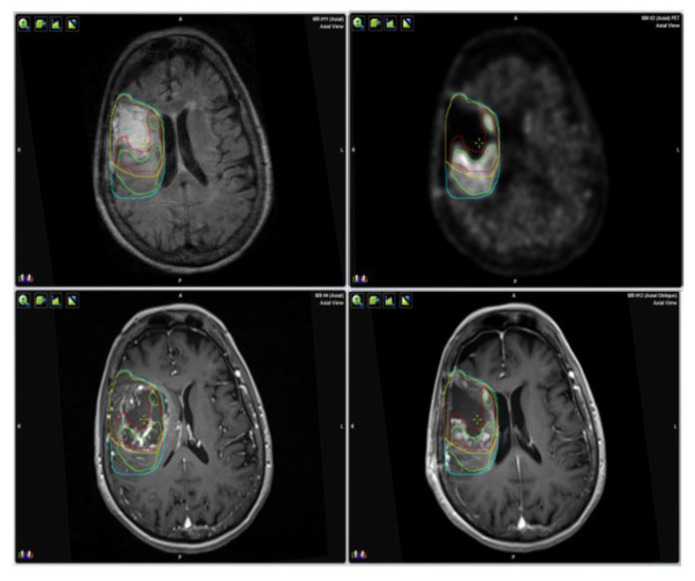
Volumes definition on post-contrast MRI, FLAIR, MRI and 11CMETPET. Clinical target volume 1 (CTV1)—yellow line; clinical target volume 2 (CTV2)—light blue; contrast-enhanced residual tumor volume (CERTV)—red; 11METPET uptake—green.
